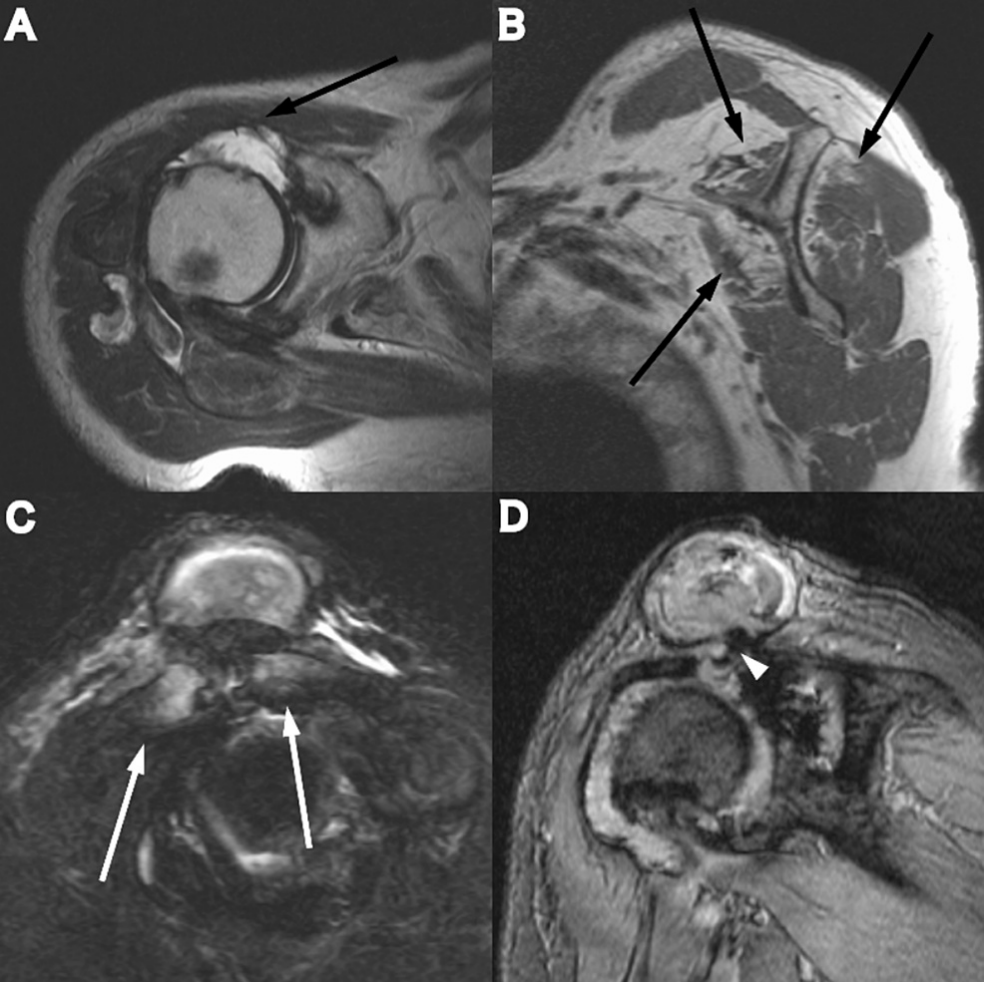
Magnetic resonance images A massive rotator cuff tear with atrophy of the supraspinatus, infraspinatus, and subscapularis muscles (black arrow) (A, B), and osteoarthritis of the acromioclavicular joint (white arrow) (C). The cyst communicated directly with the markedly degenerated glenohumeral joint (Geyser sign) on the T2-weighted MRIs (arrow head) (D).
